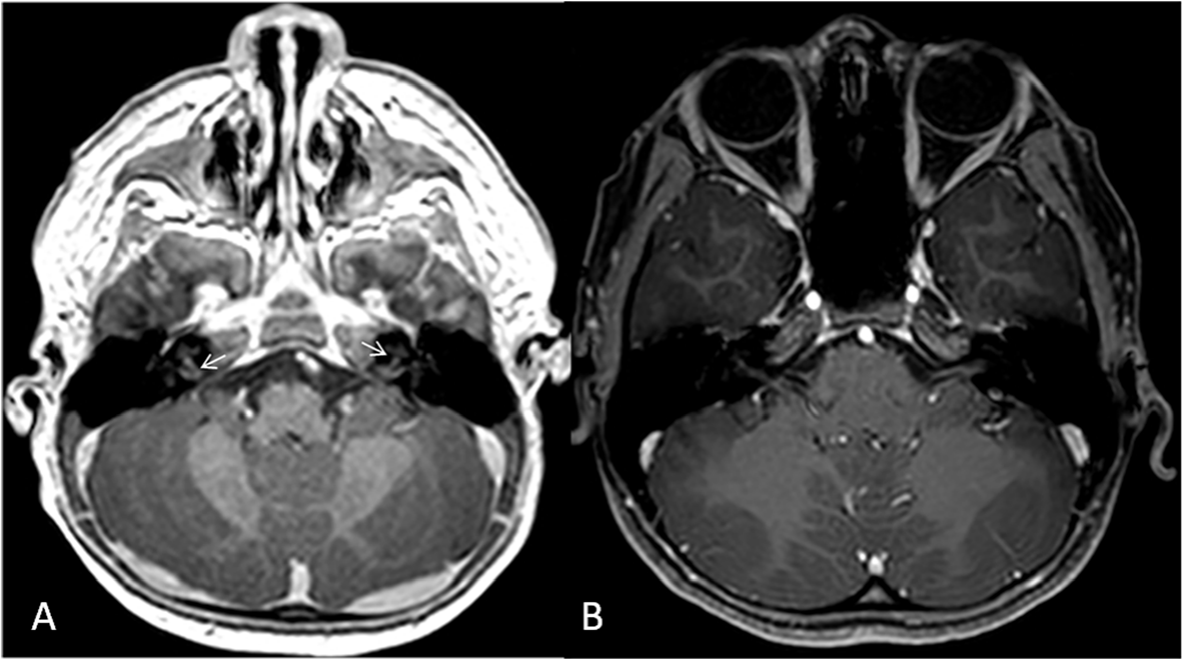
A 1-year-old girl newly diagnosed with neonatal onset multisystem inflammatory disease (NOMID), first presenting with recurrent fever and urticarial rash. MRI of the brain including internal auditory canals was performed as part of the assessment. a Selected axial 3D T1- post intravenous gadolinium administration demonstrates subtle enhancement of the cochlear nerves bilaterally (arrows). b Enhancement resolved in 6 years follow up after treatment with anakinra
